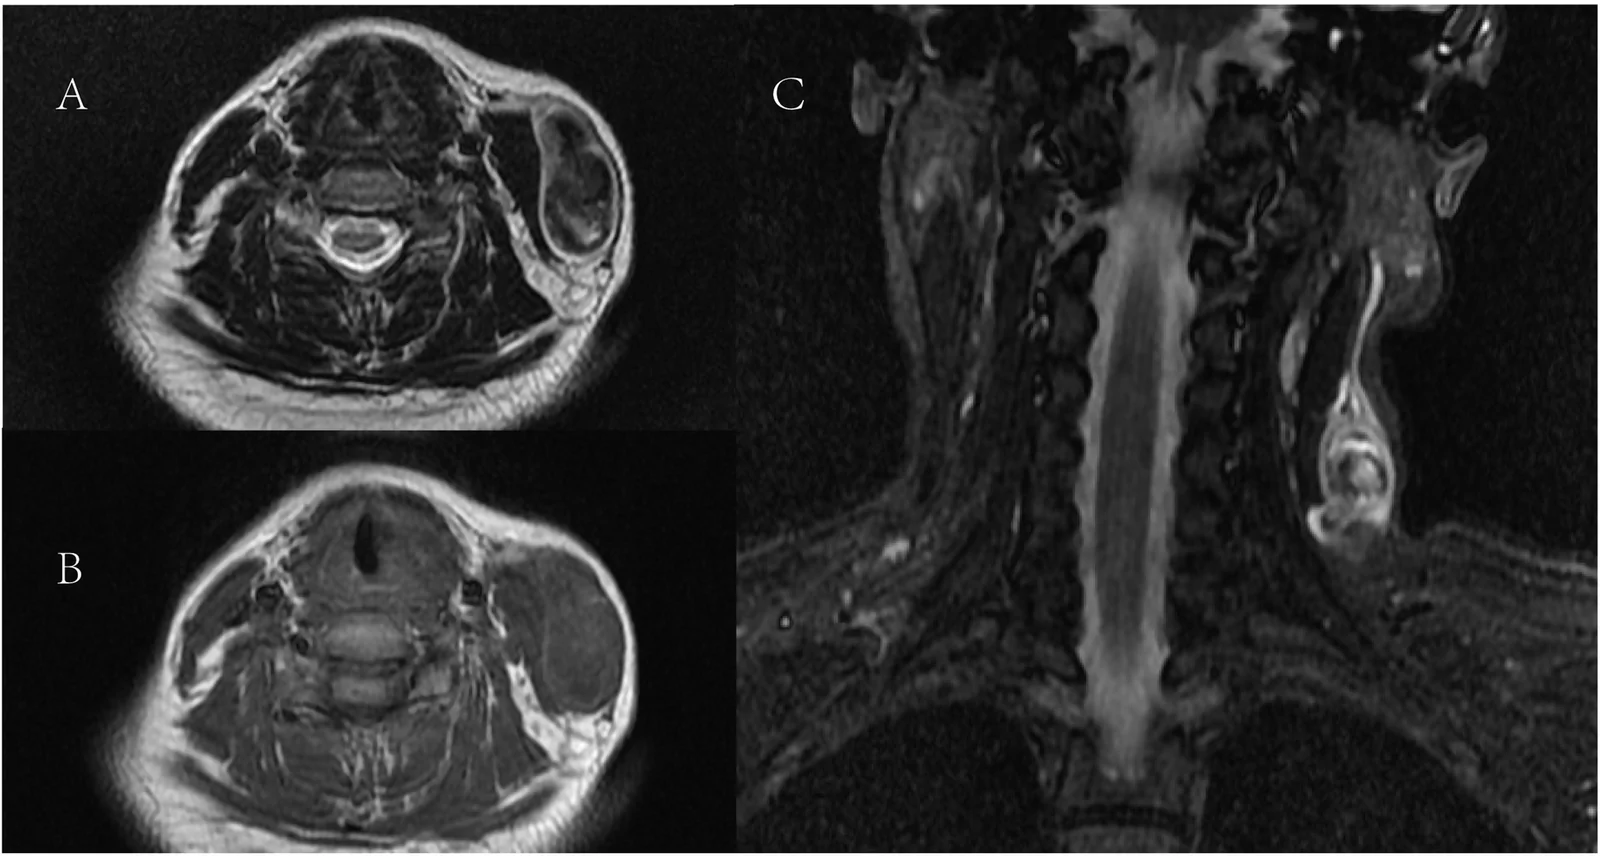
Pre-operative the MRI scan findings of the neck soft tissue. A: Axial T2; B: Axial T1; C: Coronal T2 STIR.
